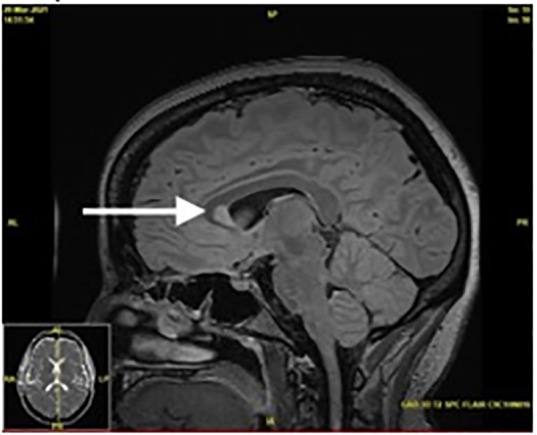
Sagittal T2-weighted magnetic resonance imaging showing periventricular hyperintensity characteristic of multiple sclerosis.
